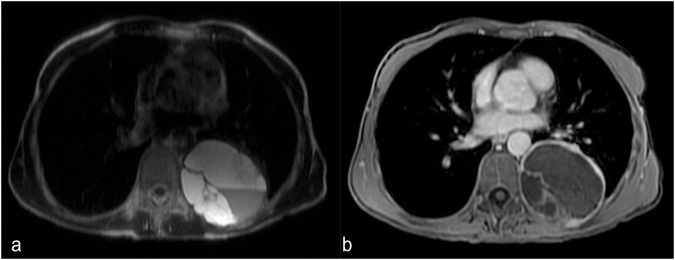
Magnetic resonance is a promising imaging modality to confirm neurogenic tumors (like paragangliomas)—the internal fluid produces a hyperintense signal on T2-weighted MRI images (a). b A typical hypointense aspect on the T1-weighted image
